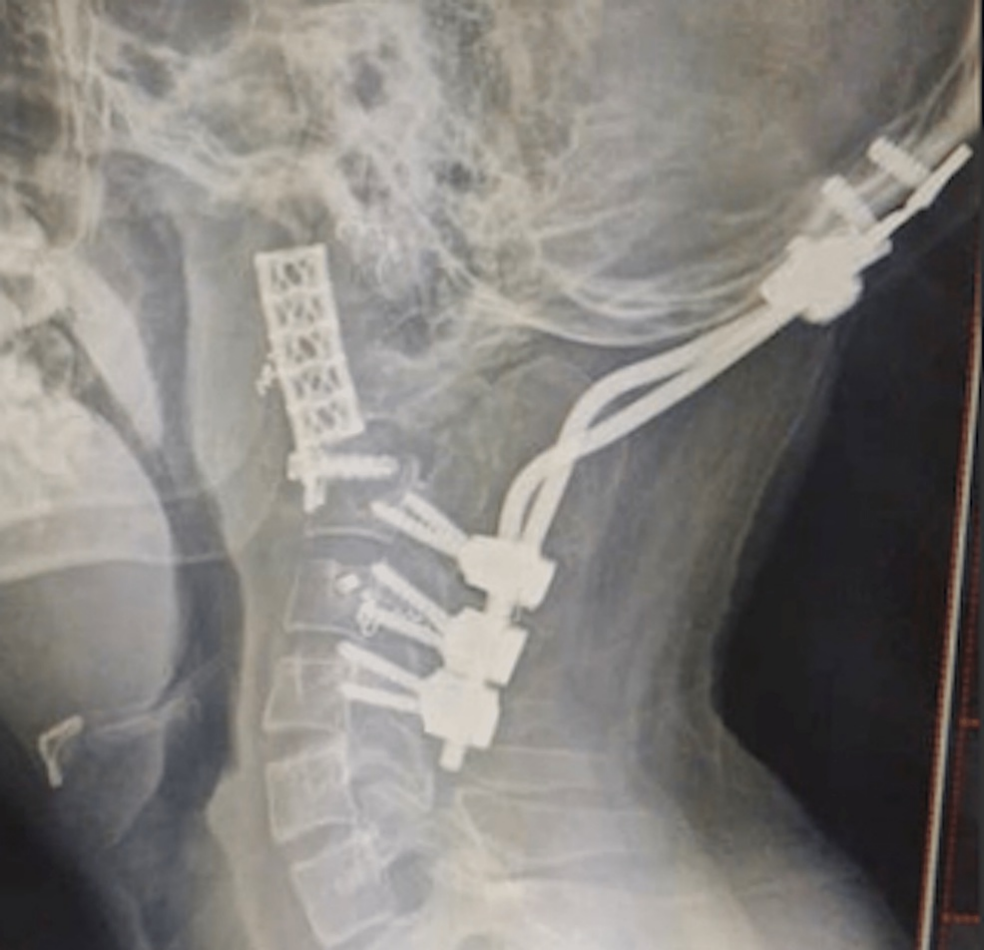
Post-operative lateral view X-ray After anterior excision of C2, the anterior column was stabilized with a mesh cage, with a screw inserted through the cage into the C3 vertebra body.
